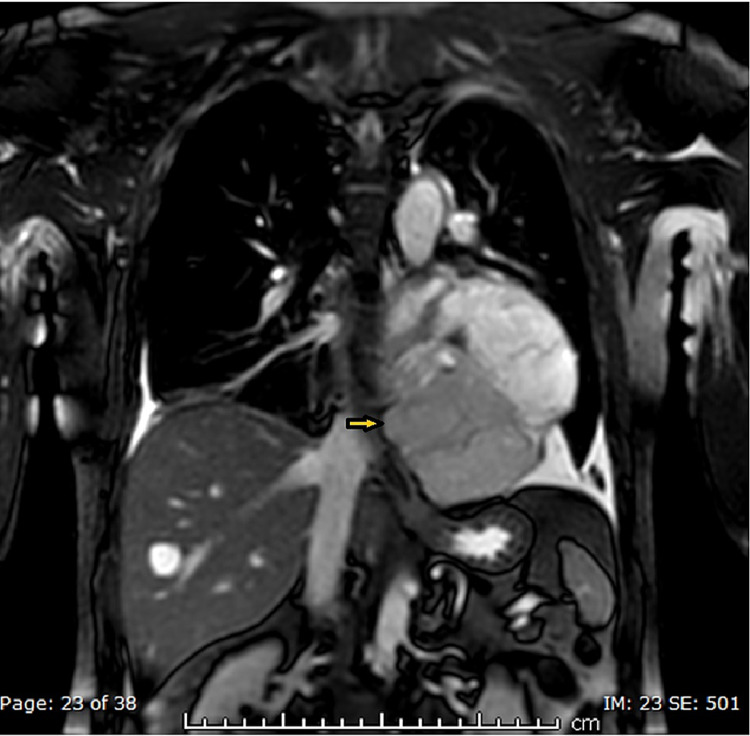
MRI-STIR sequence coronal image showing a pericardial mass lesion (arrowhead) STIR: short-TI inversion recovery
